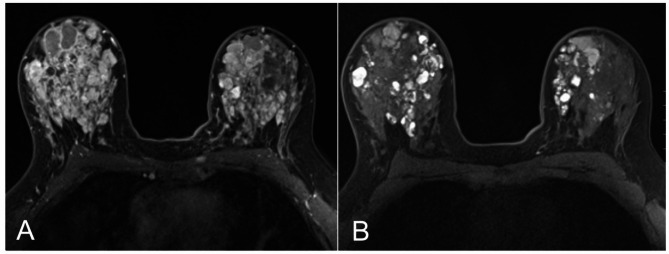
Ultrasound (A) and MRI (B) of bilateral breasts
